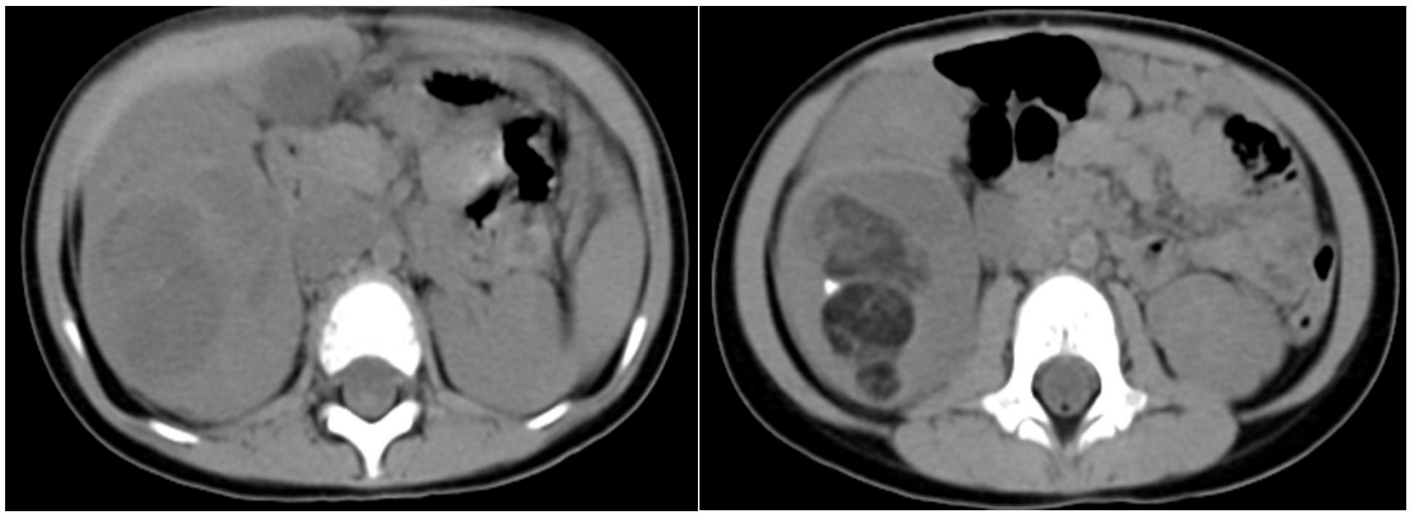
Findings in a 38-month-old girl presenting with abdominal pain for 1 week. Contrast-enhanced abdominal CT images revealed a heterogeneous mass in the middle and lower portions of the right kidney (8 cm × 8 cm × 9 cm) with imaging findings consistent with fat or hair.
